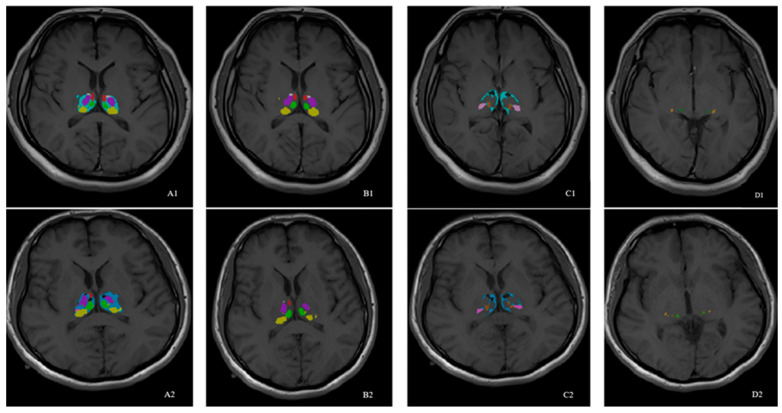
Representative axial MRI slices showing thalamic nuclei segmentations. (A1,B1,C1,D1) An epilepsy patient; (A2,B2,C2,D2) a healthy control. (A1,A2) General view of the thalamic nuclei. (B1,B2) Red: Anterior Ventral Nucleus; White: Ventral Lateral Anterior Nucleus; Purple: Ventral Lateral Posterior Nucleus; Yellow: Pulvinar Nucleus; Light green: Mediodorsal Nucleus. (C1,C2) Turquoise: Intermediate Space Nucleus; Black: Ventral Anterior Nucleus; Pink: Ventral Posterior Lateral Nucleus; Brown: Centromedian Nucleus. (D1,D2) Orange: Lateral Geniculate Nucleus; Green: Medial Geniculate Nucleus. The segmentation was performed using the VolBrain platform.
